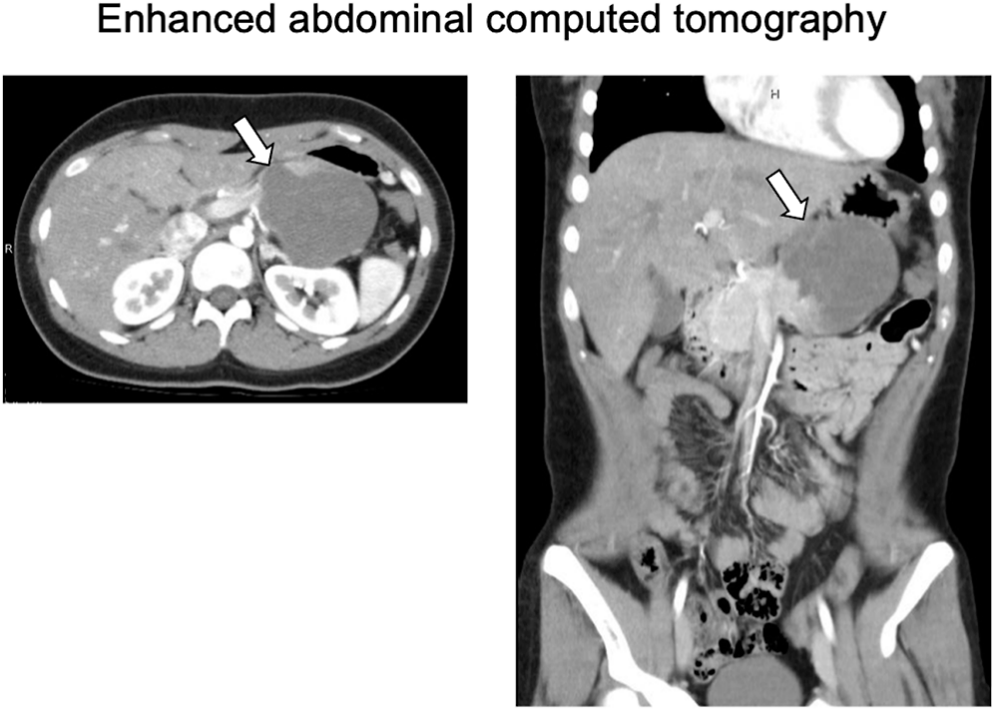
Abdominal enhanced computed tomography (CT): enhanced CT revealed an 8 cm diameter tumor, which included a pseudopapillary and solid area with a hemorrhagic necrosis lesion.
